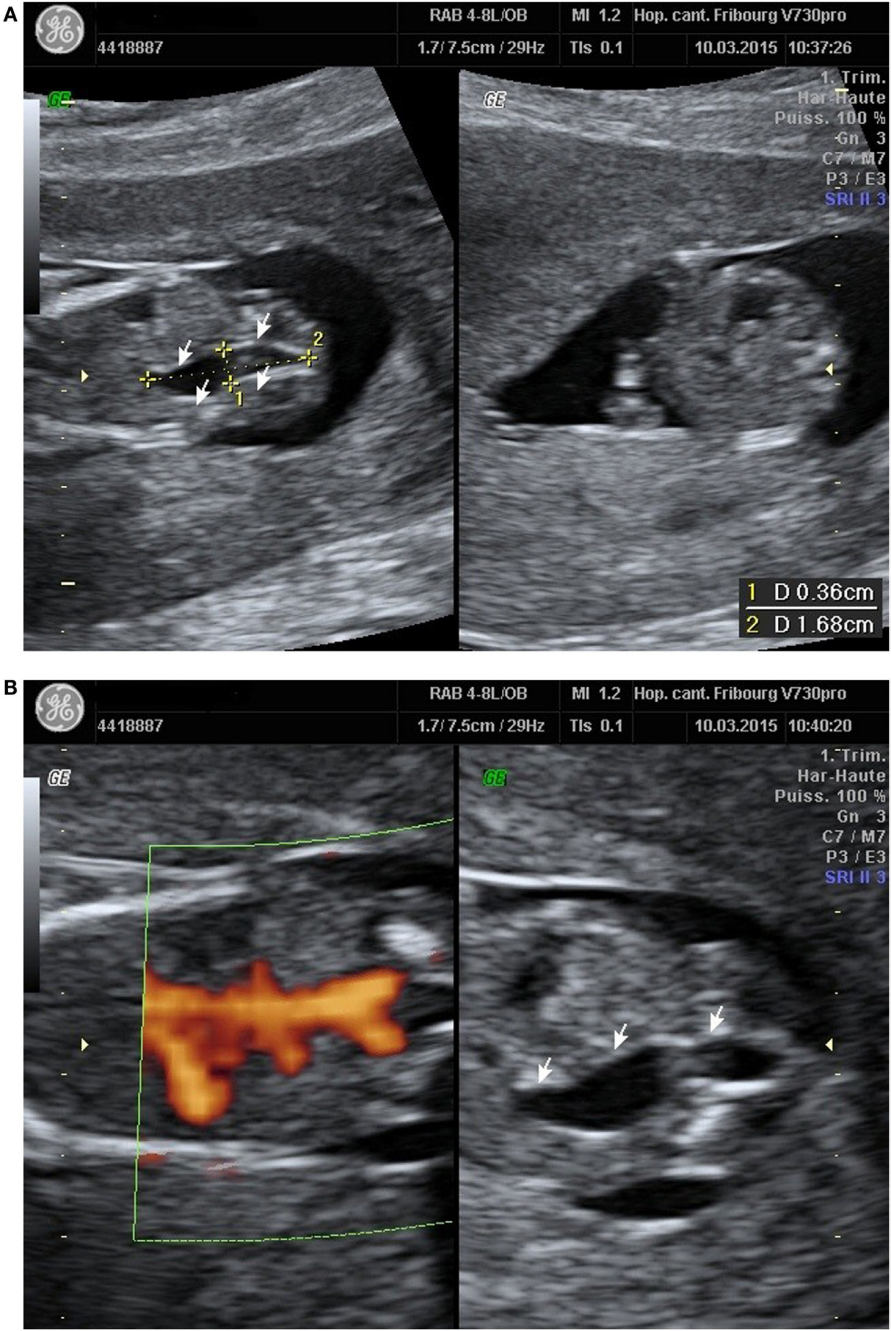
(A) Right ureteral dilatation. (B) Doppler renal arteries.
